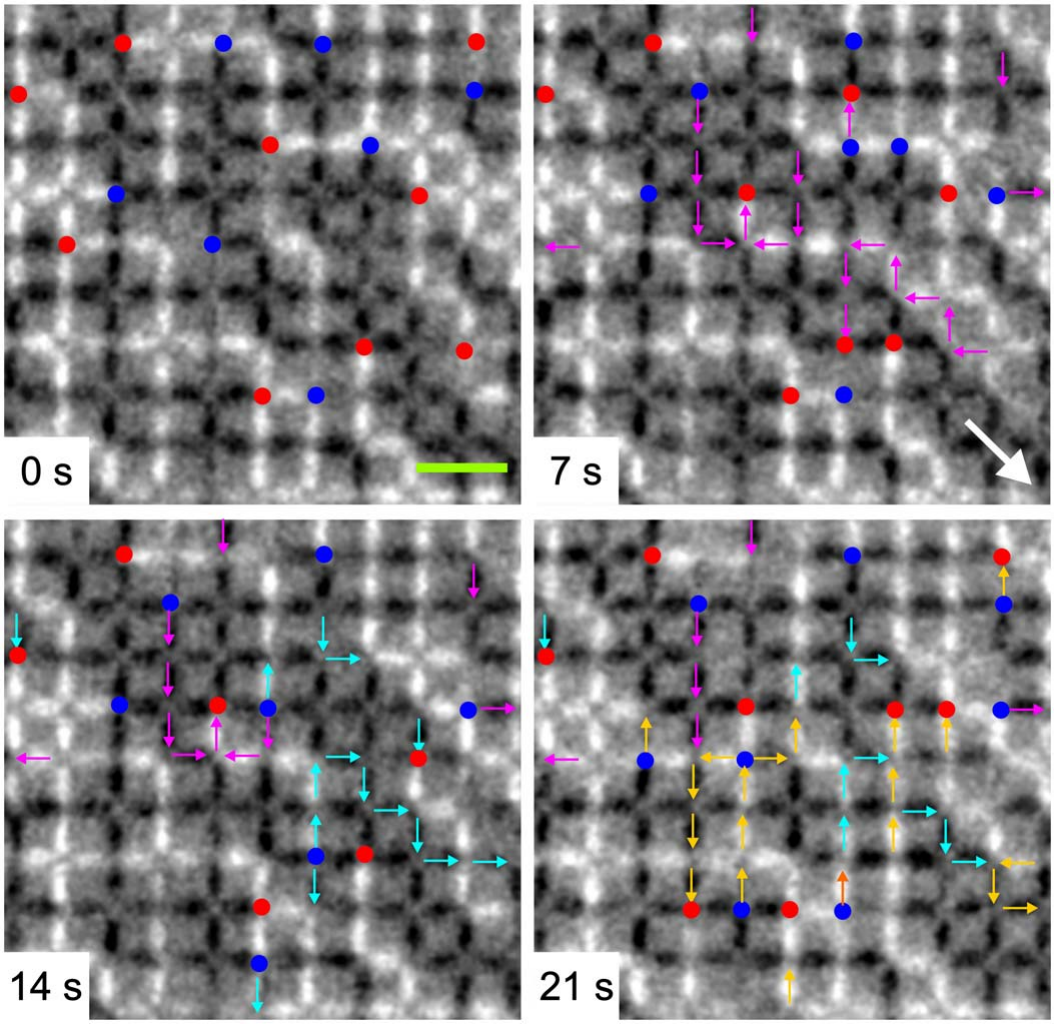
Temporal evolution of emergent magnetic monopoles. XMCD image sequence (recorded at *T* = 190 K) highlighting the thermally driven motion of emergent magnetic monopole defects (blue dots: *Q* = −2*q*, red dots: *Q* = +2*q*) in two-dimensional artificial square ice with a height offset *h* = 145 nm. Arrows of different colors (magenta, cyan blue, and yellow) indicate sequential changes in moment configurations at each instant of time (7, 14, and 21 s). The green bar and the big white arrow indicate a length of 1 μm and the incoming x-ray direction, respectively.
